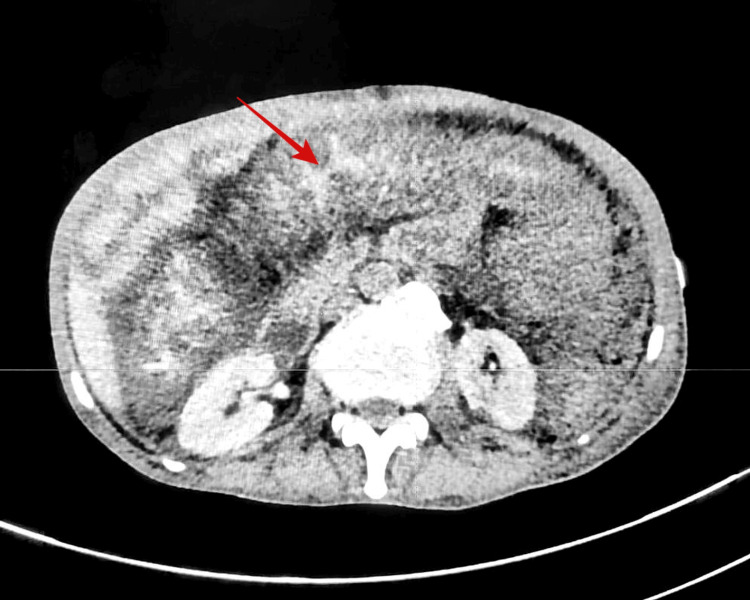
Contrast-enhanced computed tomography of the abdomen demonstrating diffuse circumferential mural thickening of the colon with mucosal hyperenhancement and submucosal edema producing the characteristic "accordion sign" (red arrow), suggestive of pancolitis.
